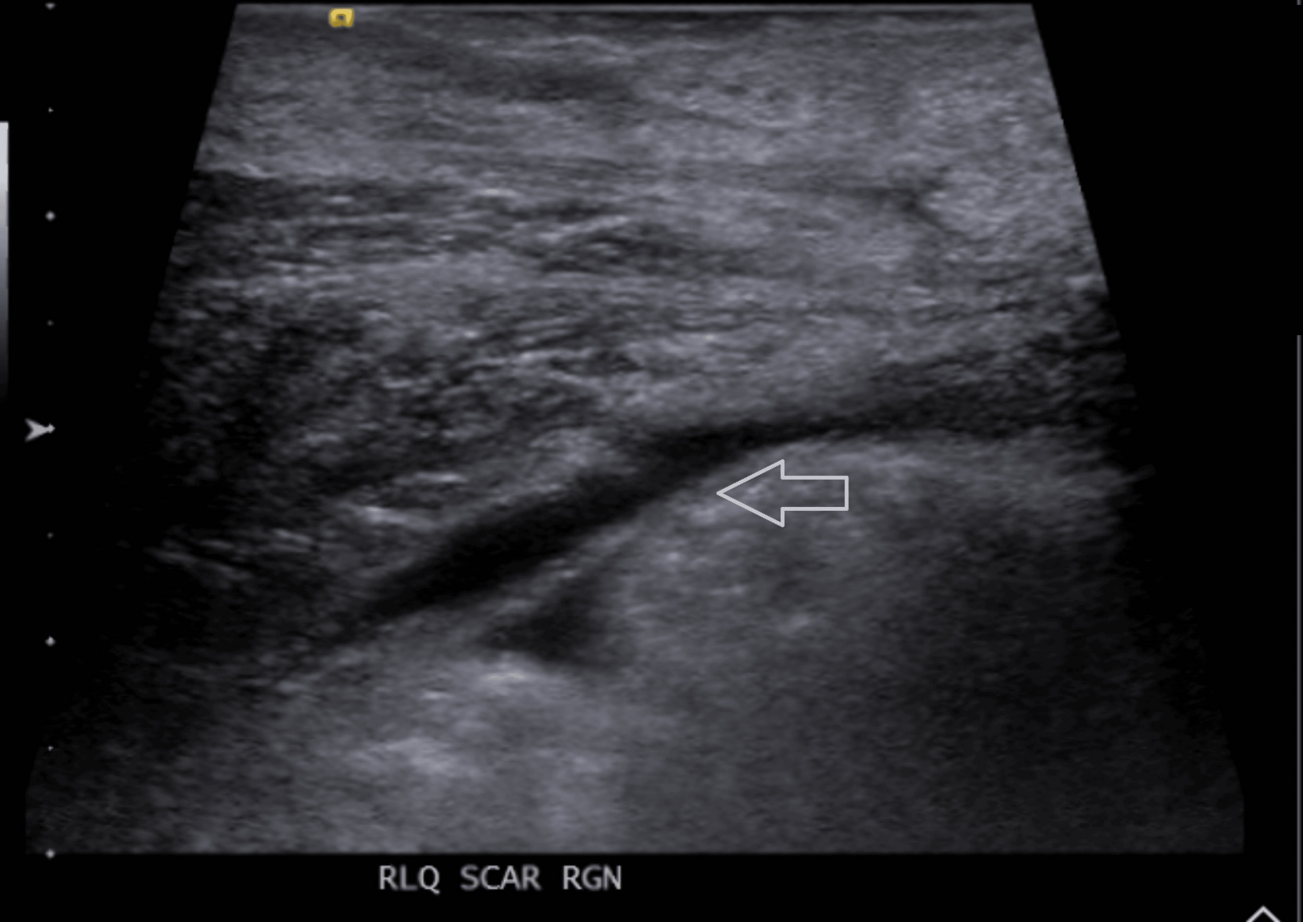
Ultrasound of the abdomen
